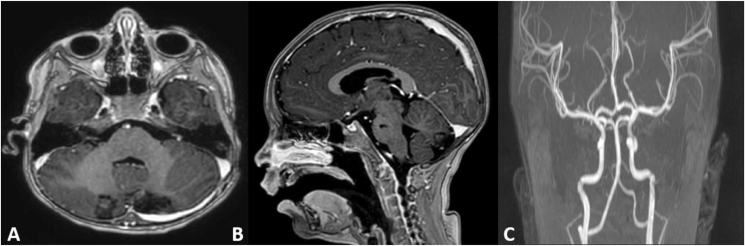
MRI brain with contrast 8 weeks follow-up showed interval resolution of previous infarcts. A: Axial view, B: sagittal view, C: Magnetic resonance angiography (MRA) showing no evidence of arterial stenosis.
